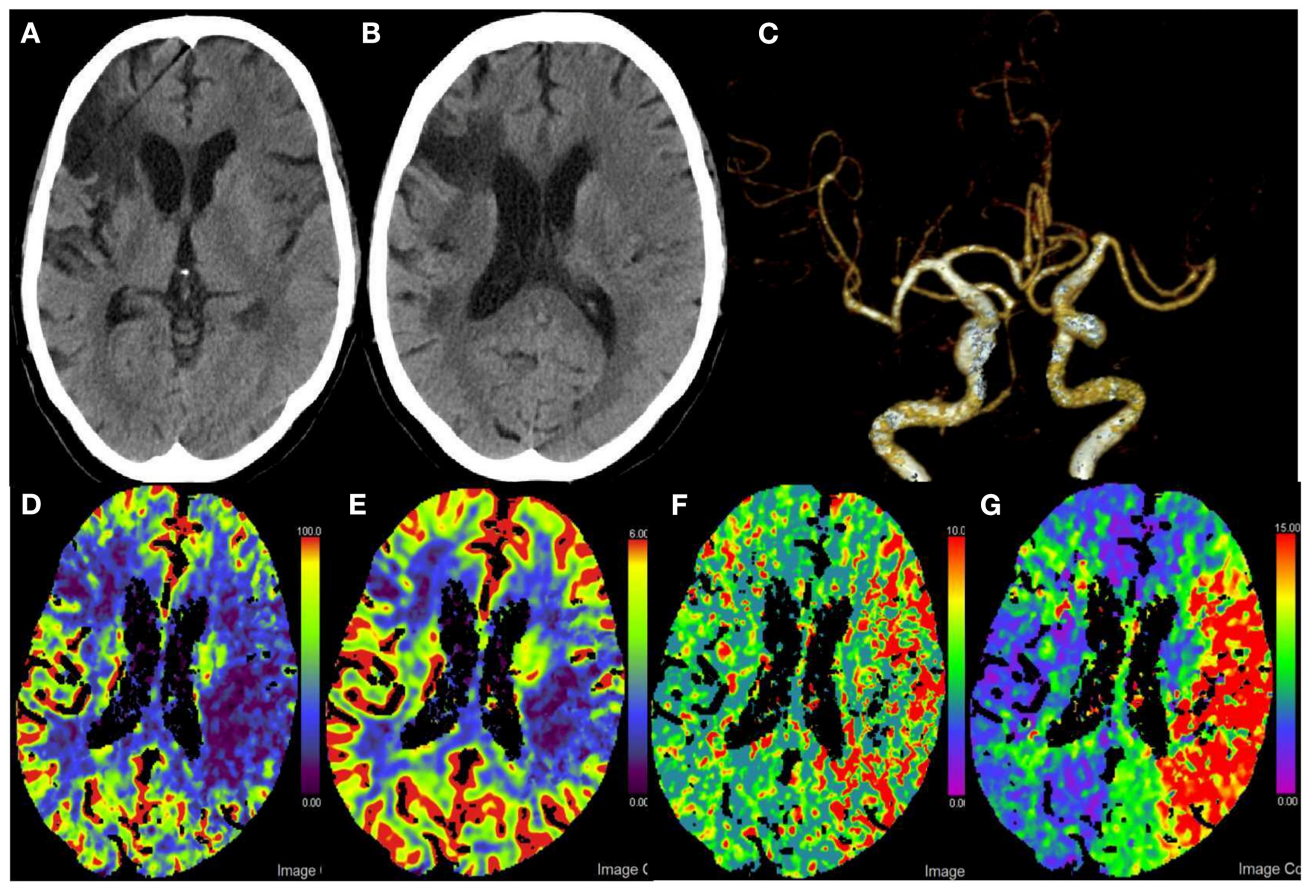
Pre-procedural imaging. (A,B) Axial pre-procedural CT of the head non-contrast shows the blurring of the gray-white border in the middle third and fourth of the left middle cerebral artery (MCA) territory. (C) Pre-procedural CT angiography demonstrating occlusion of the M1 terminus. (D–G) Pre-procedural CT perfusion shows decreased CBF and CBV and increased MTT and TTP in the left MCA territory. CBF suggests a large area of hypoperfusion in the left cerebral hemisphere; CBV suggests an infarct core that is smaller in extent than CBF, suggesting a mismatch in CBF/CBV, which may be good candidates for reperfusion therapy.
